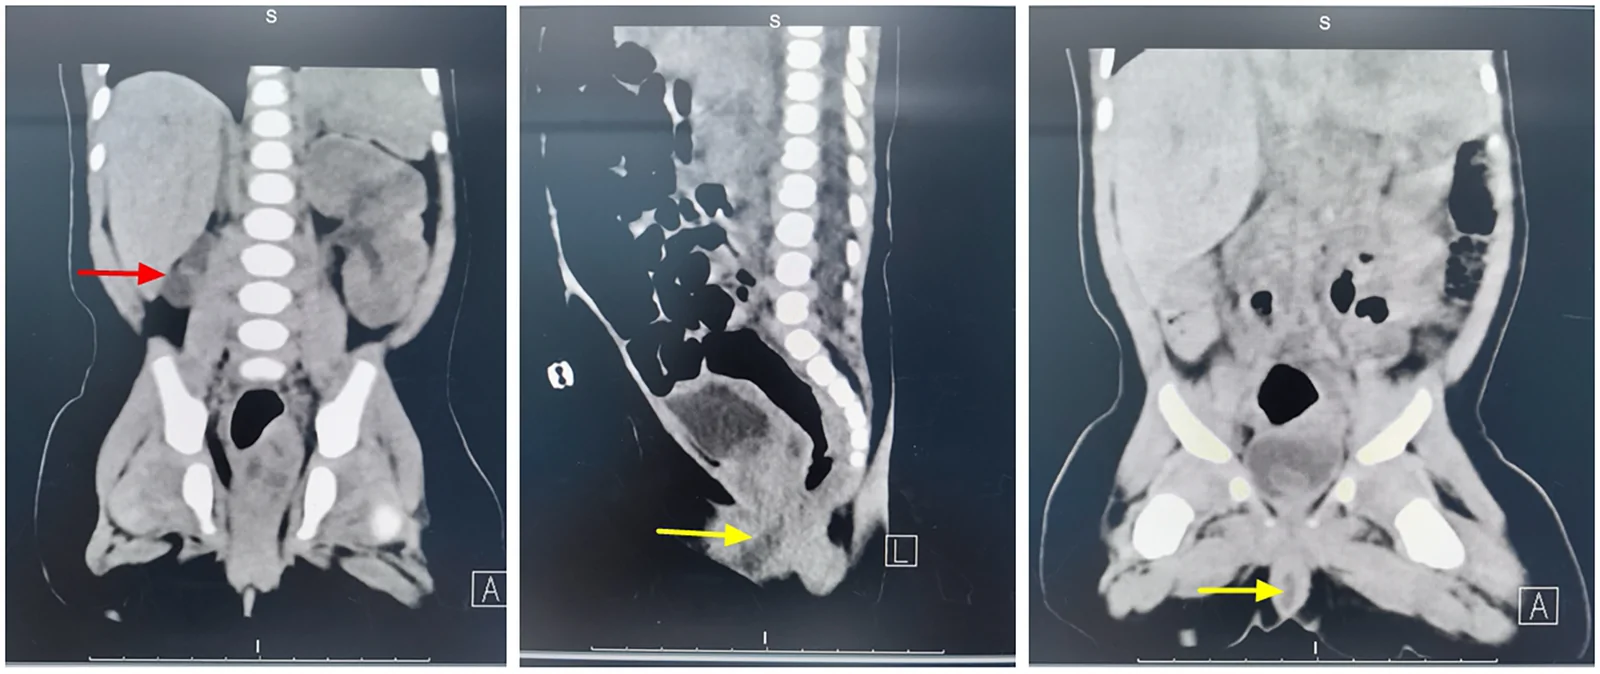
CT findings: Right side of the kidney with hypoplasia and polycystic changes (red arrow) (A); the lower segment of the uterus and cervix appear enlarged; fluid accumulation in the uterine cavity and vagina (yellow arrows) (B,C).
